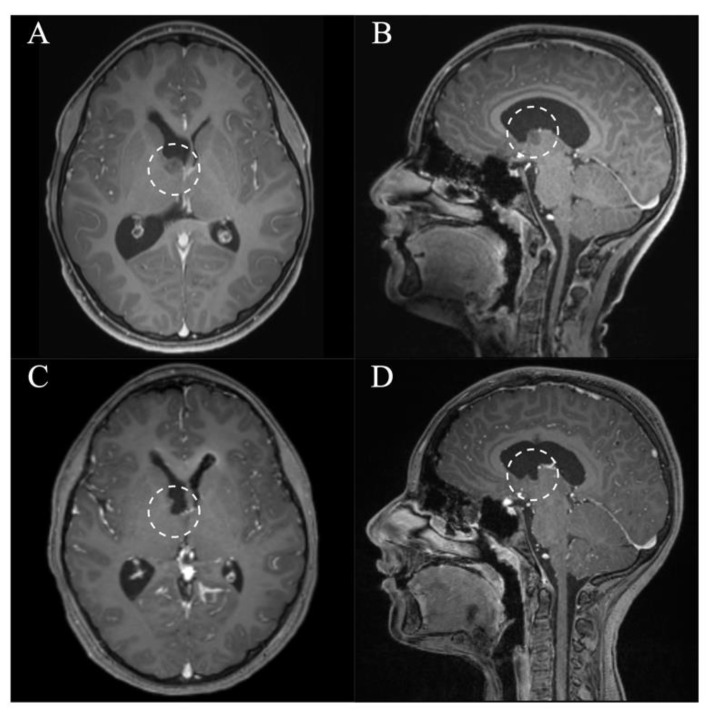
Case 8. Axial and sagittal MR images, T1-weighted sequences with contrast medium preoperatively (A,B) and immediately postoperatively (C,D) after resection of a lesion originating from the right anterior thalamus via a right frontal purely endoscopic approach. It shows a gross total resection. The lesion and resection cavity is marked with a dotted circle.
